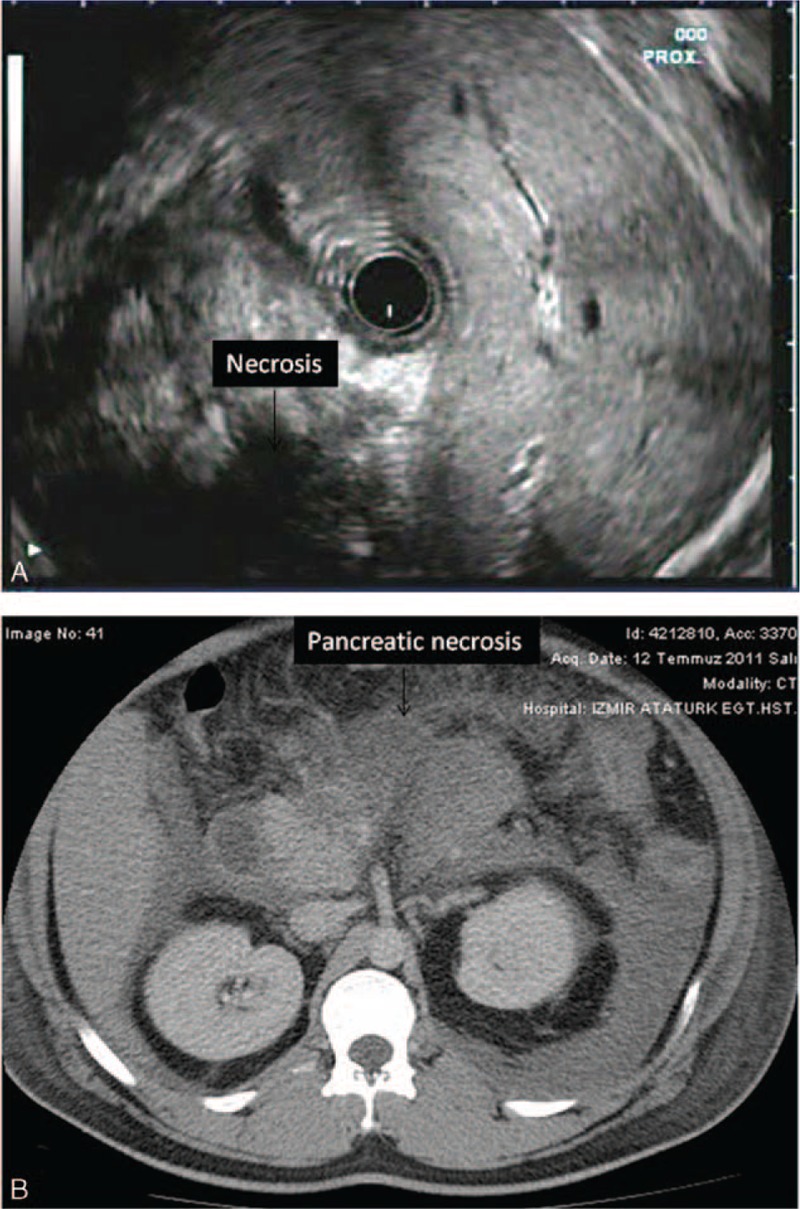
(A) Pancreatic necrosis (>30 mm) is shown. (B) CT appearance of the same patient indicating huge amount of parenchymal necrosis in the pancreas. CT = computerized tomography.
